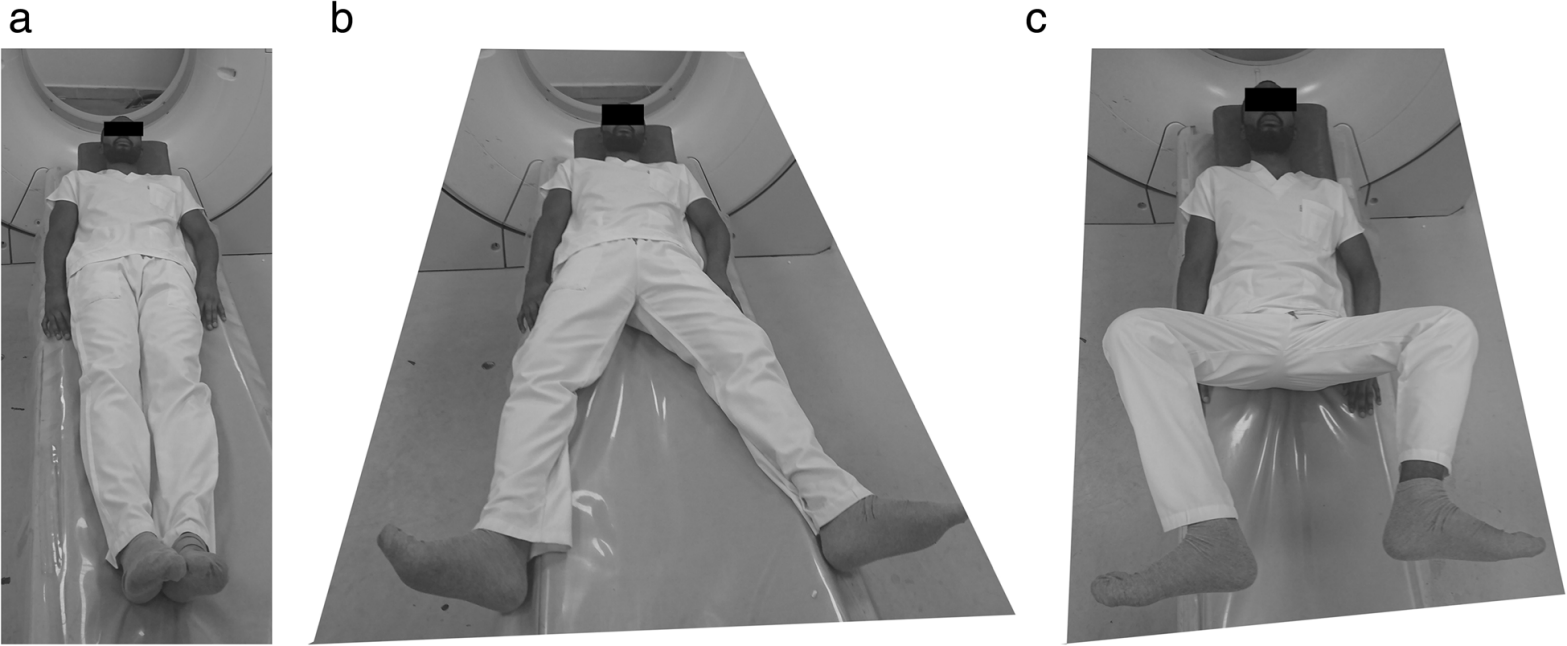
a Computed tomography imaging scans in neutral/supine. b Computed tomography imaging scans in abduction (30–45) + external rotation (20). c Computed tomography imaging scans in abduction (30–45) + external rotation (20) + abduction (30–45)
